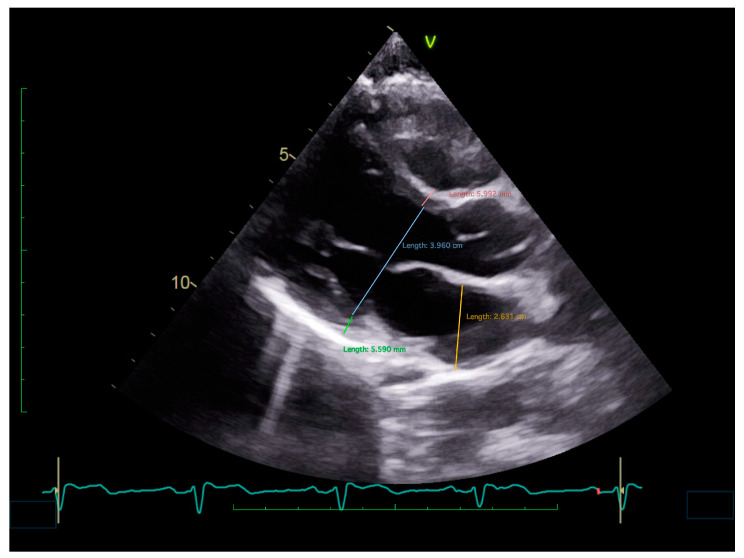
An ultrasound image of a parasternal long-axis view of the heart, showing small left cavities and thin ventricular walls in a patient with reflex syncope.
